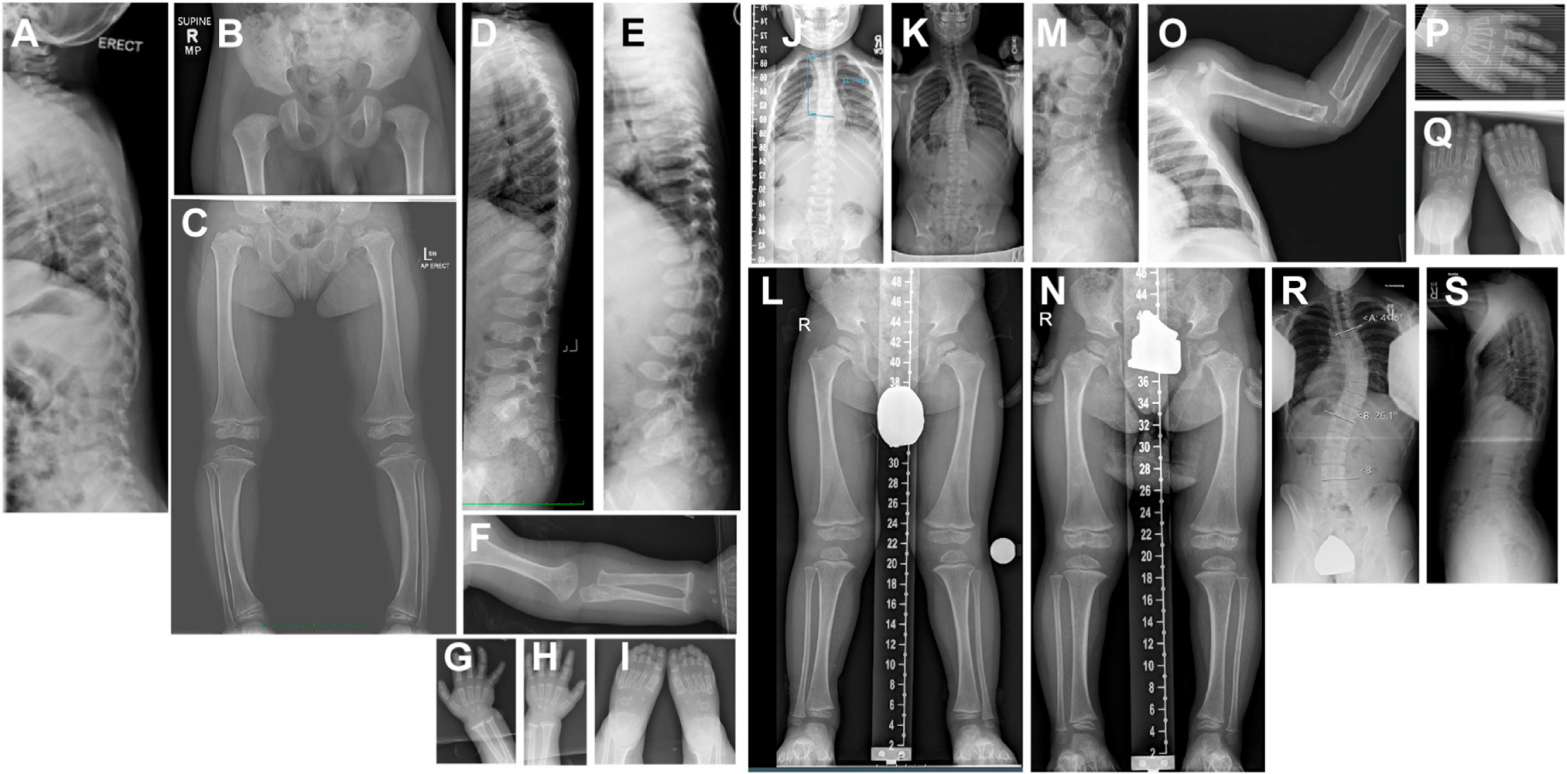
Radiographic images of probands with biallelic *SLC13A1* loss-of-function variants. A and B. Proband 1: mild anterior beaking of the lumbar vertebrae (A) and delayed ossification of the proximal capital femoral epiphyses with a stippled appearance at 2 years old (B). C and D. Proband 2 at 3 years old: tibial bowing and spondyloepiphyseal dysplasia with small and fragmented proximal capital femoral epiphyses (C) and wedge-shaped vertebral bodies (D). E-I. Proband 3 at 14 months old: thoracic kyphosis and lumbar lordosis with platyspondyly, wedging, and anterior beaking of thoracic and lumbar vertebrae (E), nonossification of the proximal humeral epiphysis with shortening of tubular bones and widened metaphyses (F-H), irregular and sclerotic capitate and hamate (G and H), and normal tarsal bones (I). J and K. Proband 3: 12° thoracic curve at 4 years old (J) with progression to S-shaped scoliosis with 28° upper thoracic (T3-T8), 19° lower thoracolumbar (T10-L3), and 11° lumbar curve at 13 years old (K). L. Proband 3 at 3 years old: valgus of bilateral knees with foreshortening of proximal femoral metaphyses and widening of the femoral and tibial metaphyses. M-P. Proband 4 at 14 months old: ovoid and bullet shaped vertebral bodies with anterior wedging (M), shorted tubular bones with widened metaphyses and fragmented proximal epiphyses (N-P), and normal appearing carpal and tarsal bones (O and P). Q. Proband 4 at 2 years old: slightly flared proximal femoral metaphyses bilaterally with relative foreshortening. R and S. Proband 5: 41° and 44° dextroconvex thoracic curve at 14 years old and 17 years old, respectively, with levoconvex lumbar scoliosis with diminished vertebral body height of multiple mid and lower thoracic bodies.
